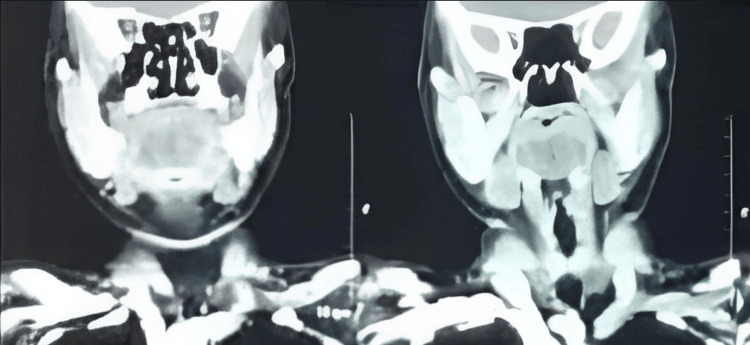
Computed tomography images on the left and right show sublingual swelling.
